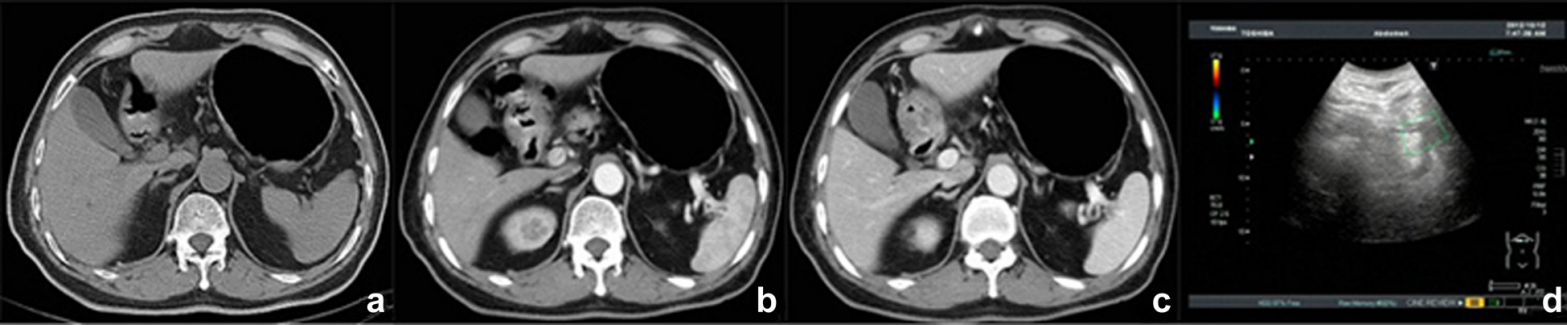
T3 gastric cancer in gastric antrum. (a-c) CT scanning. The nodules were visible around the fat space, and serosal surface was rough. (d) Ultrasound imaging. The visible lesions were invading outside the serosa.
